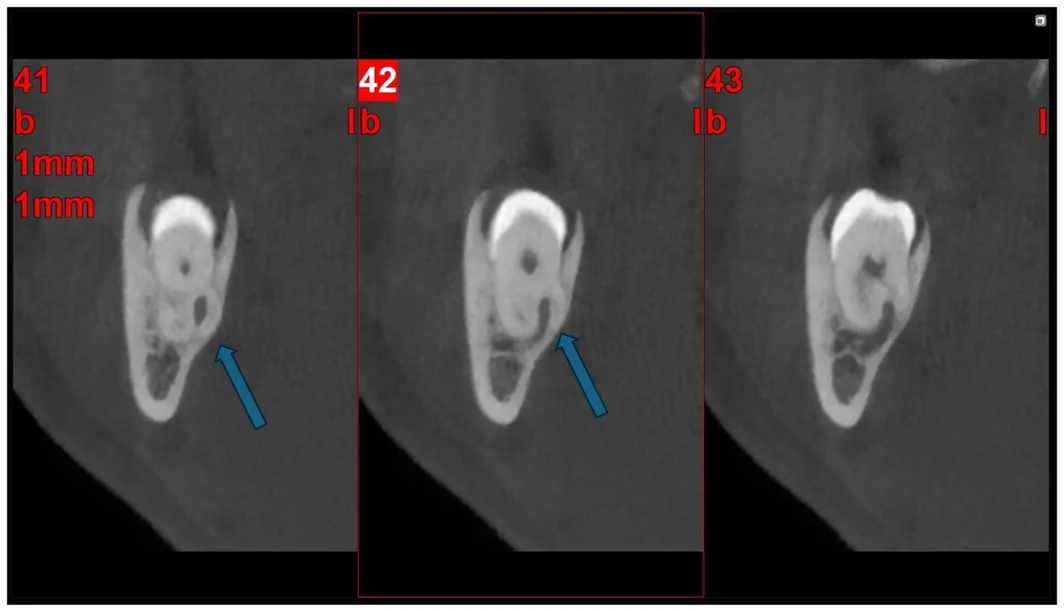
The coronal section of the CBCT shows an interradicular course of the nerve, with the dental roots fused at the apex.
